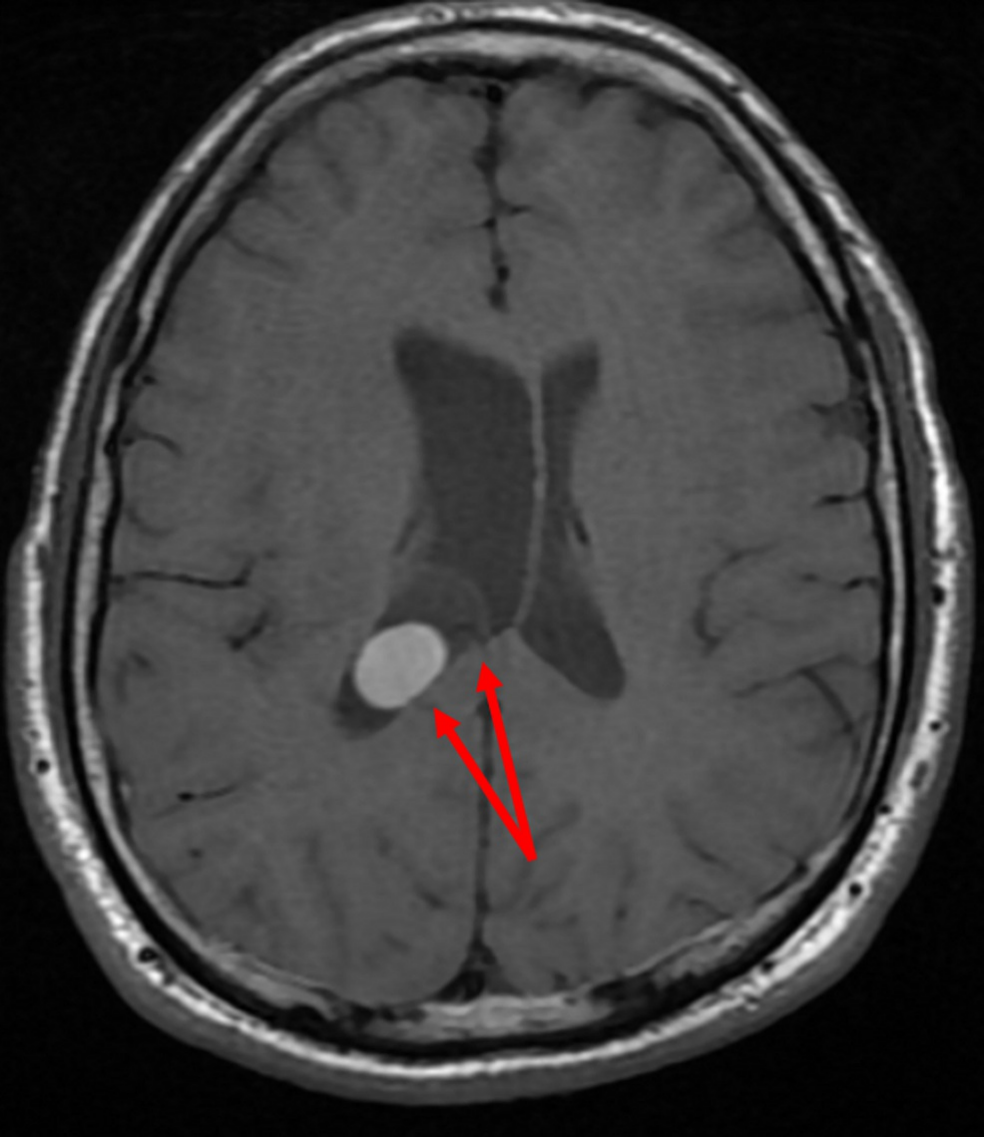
Axial T1-weighted brain MRI demonstrating hyperintense lesion within larger cystic lesion and enlargement of the right ventricle.
